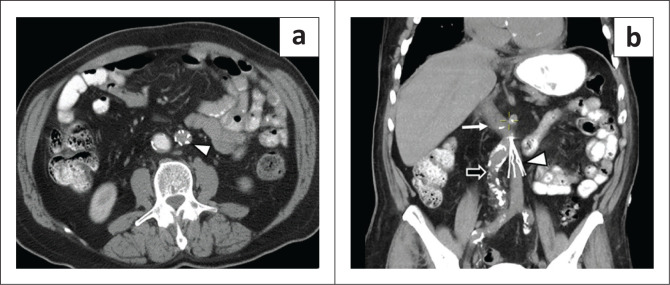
A 53-year-old male with left lower quadrant pain was found to have acute diverticulitis with abscess formation. (a) Contrast enhanced axial CT abdomen image showed a left-sided inferior vena cava (IVC) with a filter (arrowhead). Knowledge of the anatomy was mandatory prior to IVC filter placement in this case. (b) Coronal CT abdomen of same patient shows the IVC to the left of the atherosclerotic aorta (open arrow). The left IVC then turns towards right at the level of left renal vein (not shown) to continue as the normal right suprarenal IVC (white thin arrow).
